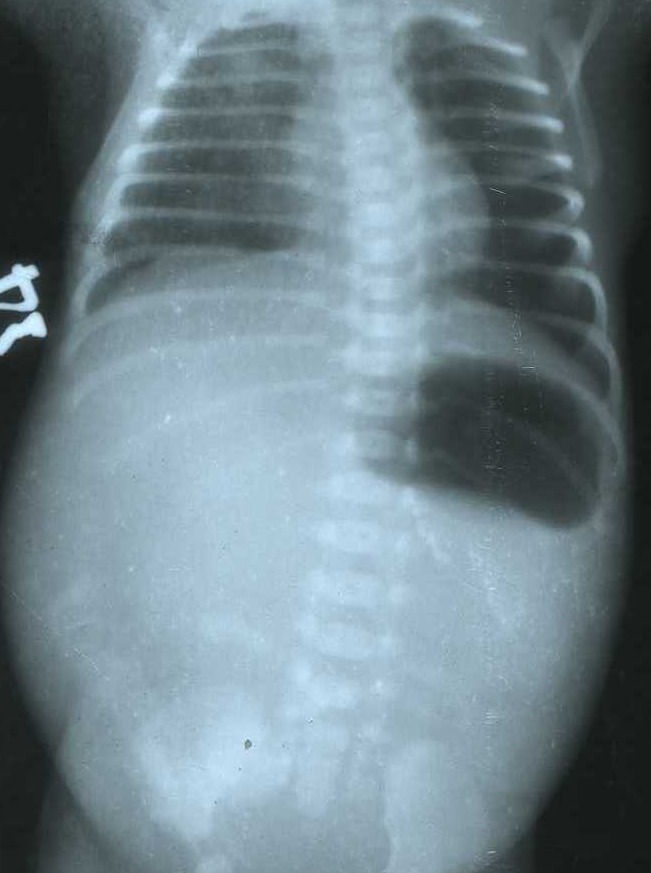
Figure 1: A plain X-ray of the abdomen showing a single large gastric air bubble with no gas distally.
